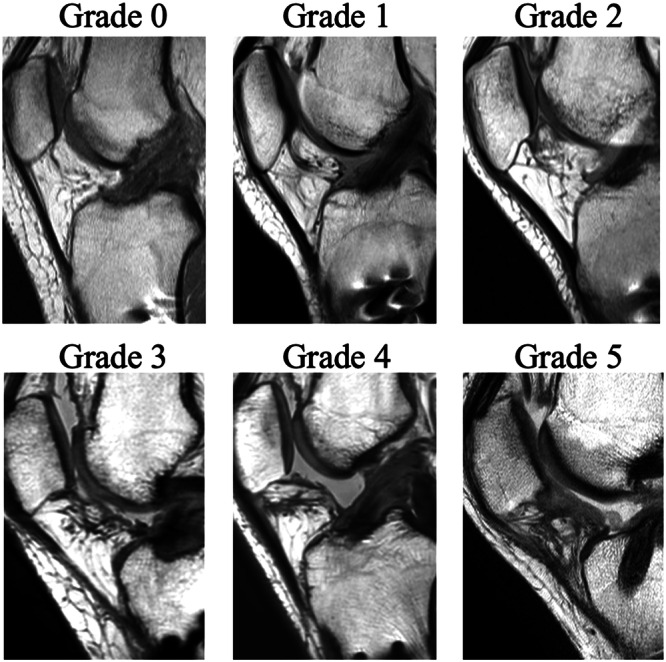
Proton density sagittal magnetic resonance image (MRI) classifying IFP fibrosis from Grade 0 to Grade 5. The definitions are as follows; Grade 0, no fibrosis; Grade 1, focal fibrosis; Grade 2, between Grades 1 and 3; Grade 3, complete band fibrosis from the lower pole of the patella to the tibial plateau; Grade 4, between Grades 3 and 5; Grade 5, diffuse and infiltrated fibrosis (occupying more than 75% of the area).
